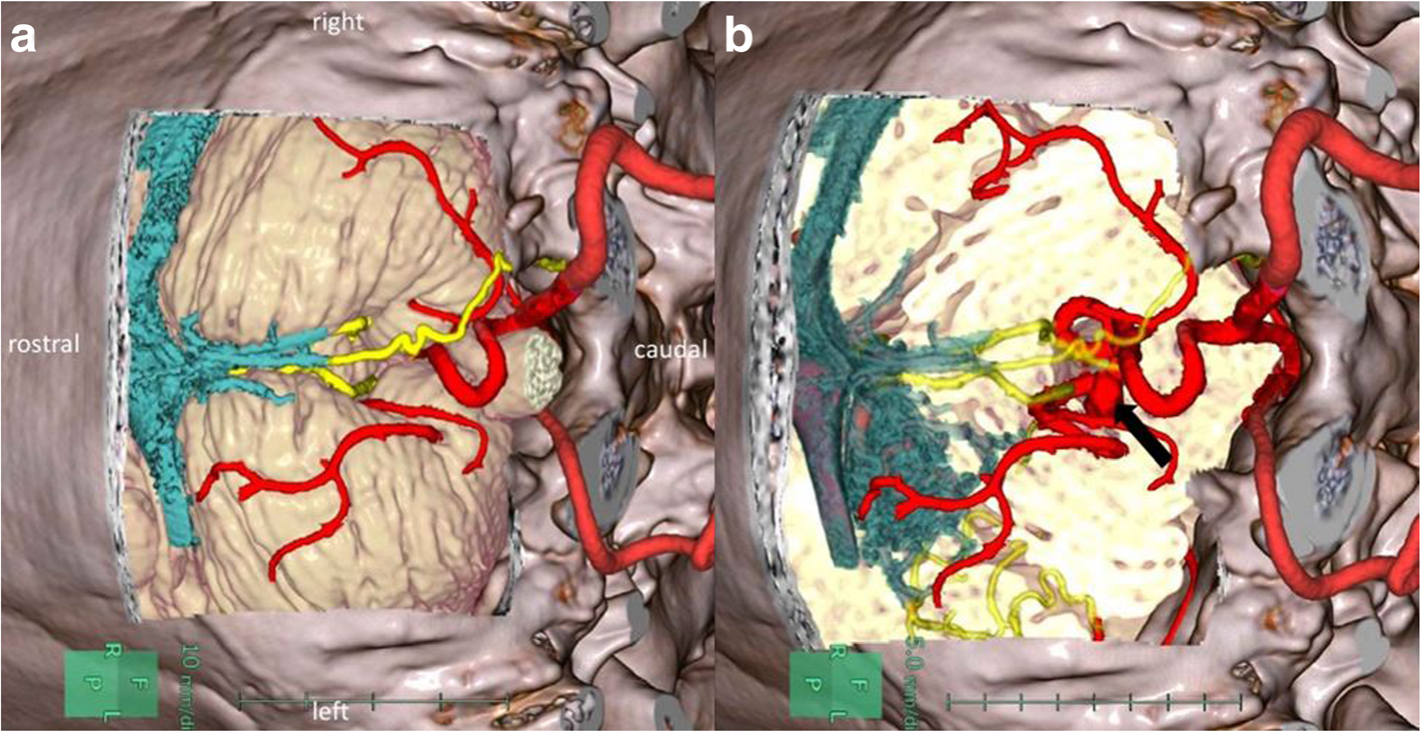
Reconstructed three-dimensional computed tomography angiography and venography. a, b Three-dimensional reconstructed images, showing the vertebral artery and PICA (red), distal posterior inferior cerebellar artery aneurysm(DPICAAn) (arrow), one feeder from the right PICA of the AVM (yellow), and nidus–drainers–transverse sinus (blue)
